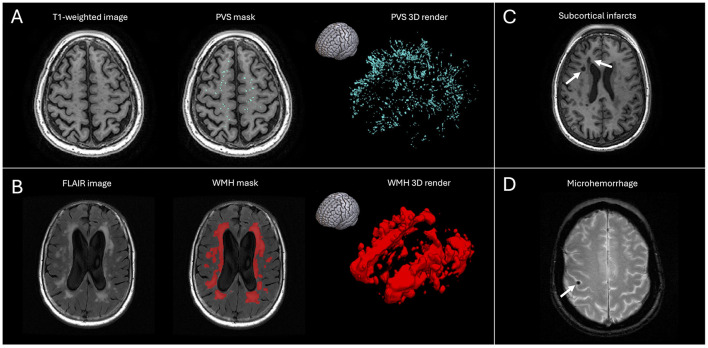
Representative images of cerebral small vessel disease radiographic markers assessed in this study. (A) Axial T1-weighted image without and with overlaid PVS mask in cyan. The image on the right is the corresponding 3D rendering of the PVS masks; the brain icon indicates the orientation of the 3D rendering. (B) Axial FLAIR image without and with overlaid WMH mask in red. The image on the right is the corresponding 3D rendering of the WMH mask; the brain icon indicates the orientation of the 3D rendering. (C) Axial T1-weighted image at baseline of a participant with subcortical infarcts (white arrows). (D) Axial T2-star image at baseline of a participant with a microhemorrhage (white arrow).
